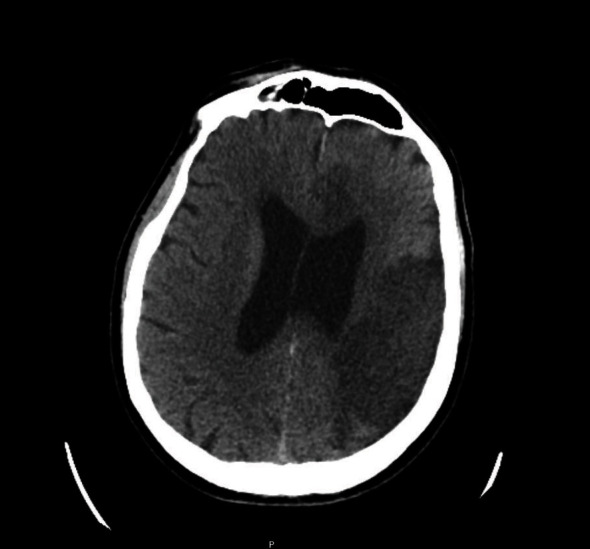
Noncontrast CT brain performed at the time of initial presentation, revealing established infarcts in the left MCA and ACA territories.
